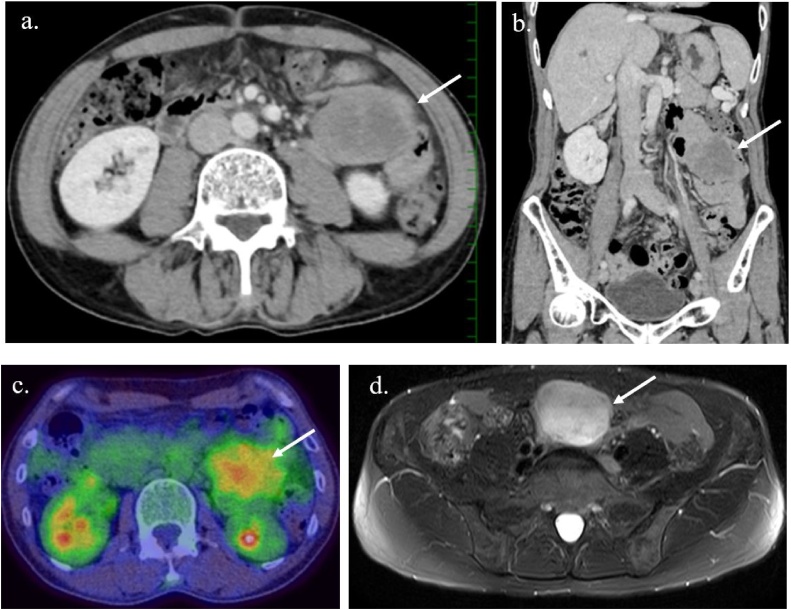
a,b: Abdominal CT indicated 5 cm, a smooth-marginated and inhomogeneous enhanced mass (white arrow). C: On PET-CT, FDG concentrated on the tumor, SUV max was 4.0. d: On MRI, the tumor showed low signal in T1-weighted images and enhanced signal in T2-weighted images. Abbreviations: CT, computed tomography; FDG, fluorodeoxryglucose; MRI, magnetic resonance imaging; PET, Positron emission tomography; SUV, standardized uptake value.
